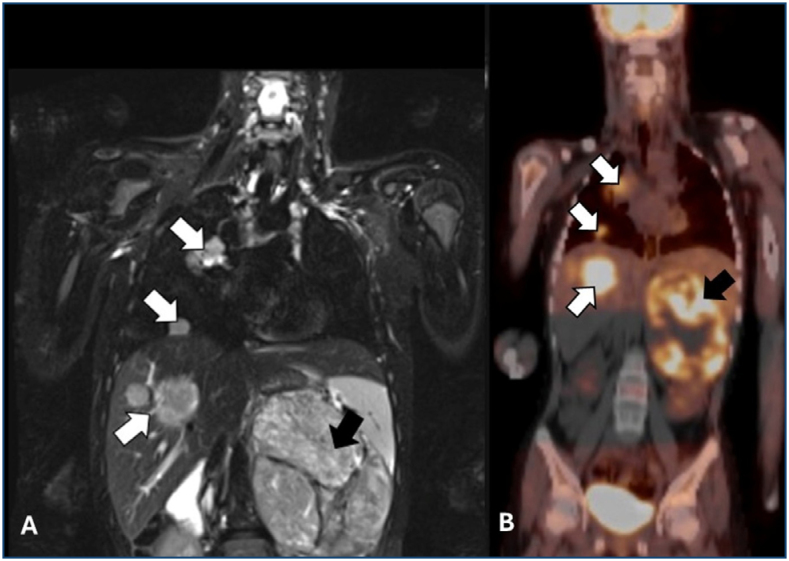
(A, B) 18-year-old girl with left adrenocortical carcinoma: Coronal whole-body magnetic resonance imaging short tau inversion recovery image (A) shows the hyperintense solid mass in the left adrenal region (black arrow) associated with multiple liver and lung metastases and hilar lymphadenopathies (white arrows). positron emission tomography-computed tomography image (B) demonstrates the same findings with increased Fludeoxyglucose F 18 uptakes (white arrows and black arrow).
